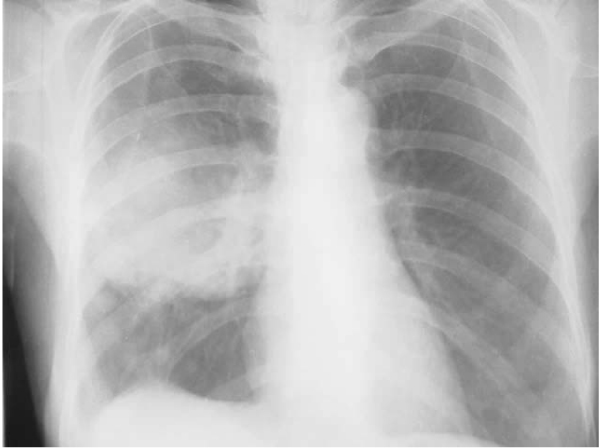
A chest roentgenogram (PA view) of a non-smoking housewife with prolonged history of ETS exposure from father and spouse showing a mass lesion in right mid-zone, which turned out to be bronchogenic squamous cell carcinoma.
